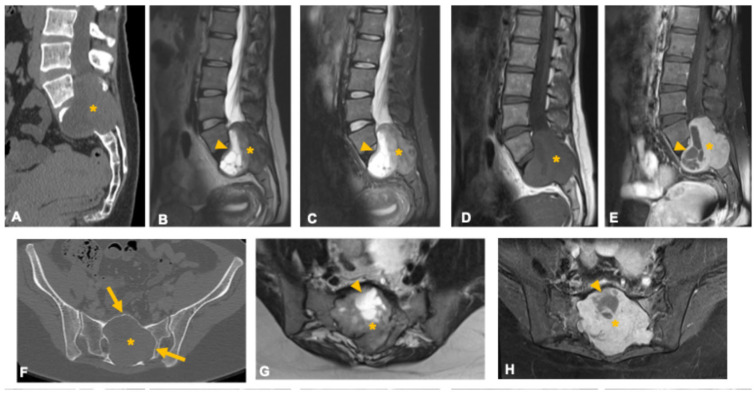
Giant invasive sacral schwannoma in a 34-year-old woman. Axial (A) and sagittal (F) CT images show a large sacral mass (asterisk) causing bone erosion and obliteration of the vertebral canal. The presence of sclerotic margins and smooth bone remodeling (arrows in (F)) suggests slow tumor growth. MRI sequences—T2-weighted (B,G), STIR (C), and T1-weighted (D) demonstrate a predominantly solid, heterogeneous mass (asterisks) with internal cystic areas (arrowheads) and clear delineation of canal and foraminal involvement. Post-contrast images (E,H) reveal intense enhancement of the solid components with non-enhancing cystic regions. Bone remodeling, extensive foraminal and canal involvement, and the presence of cystic areas support this diagnosis.
